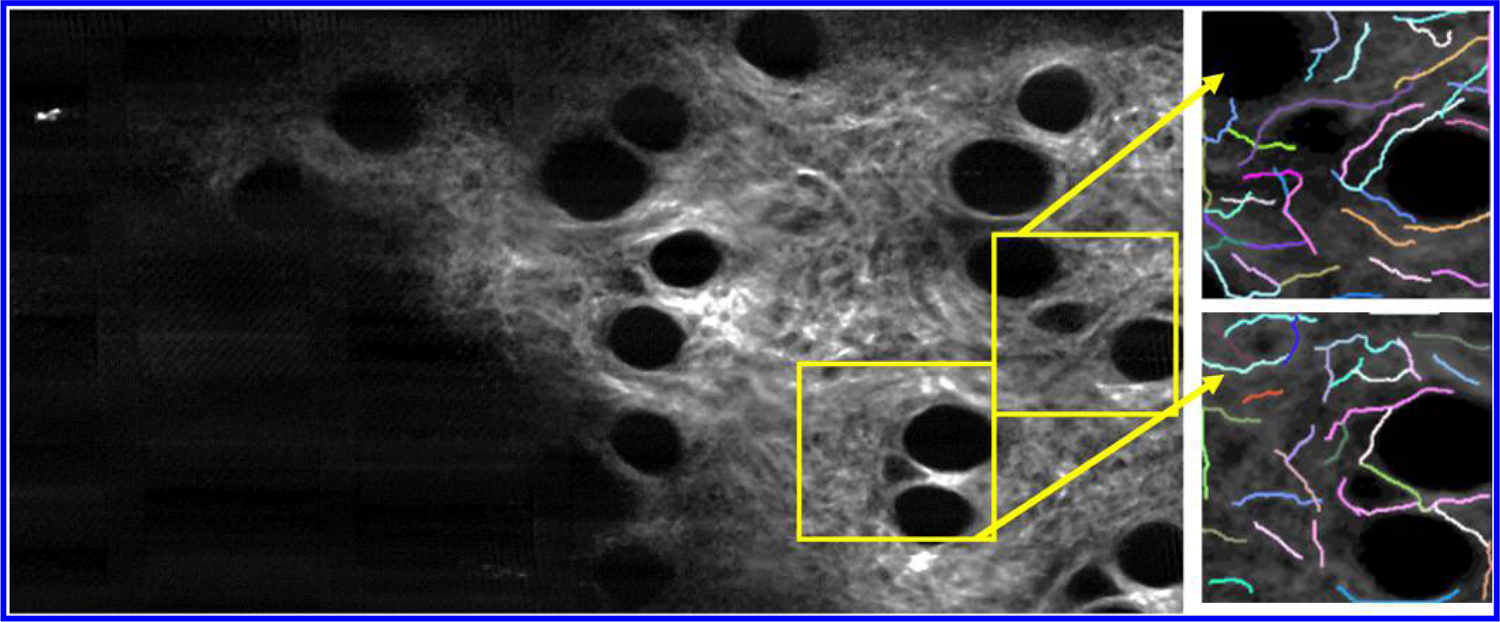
Cell-based regional SHG image tiles created by the collagen analysis module and analyzed using CT-FIRE. Selected individual collagen fibers are identified and the associated orientation angles can be calculated. The blank region at the lower left corner in the left image is associated with the inside of the skin wound.
